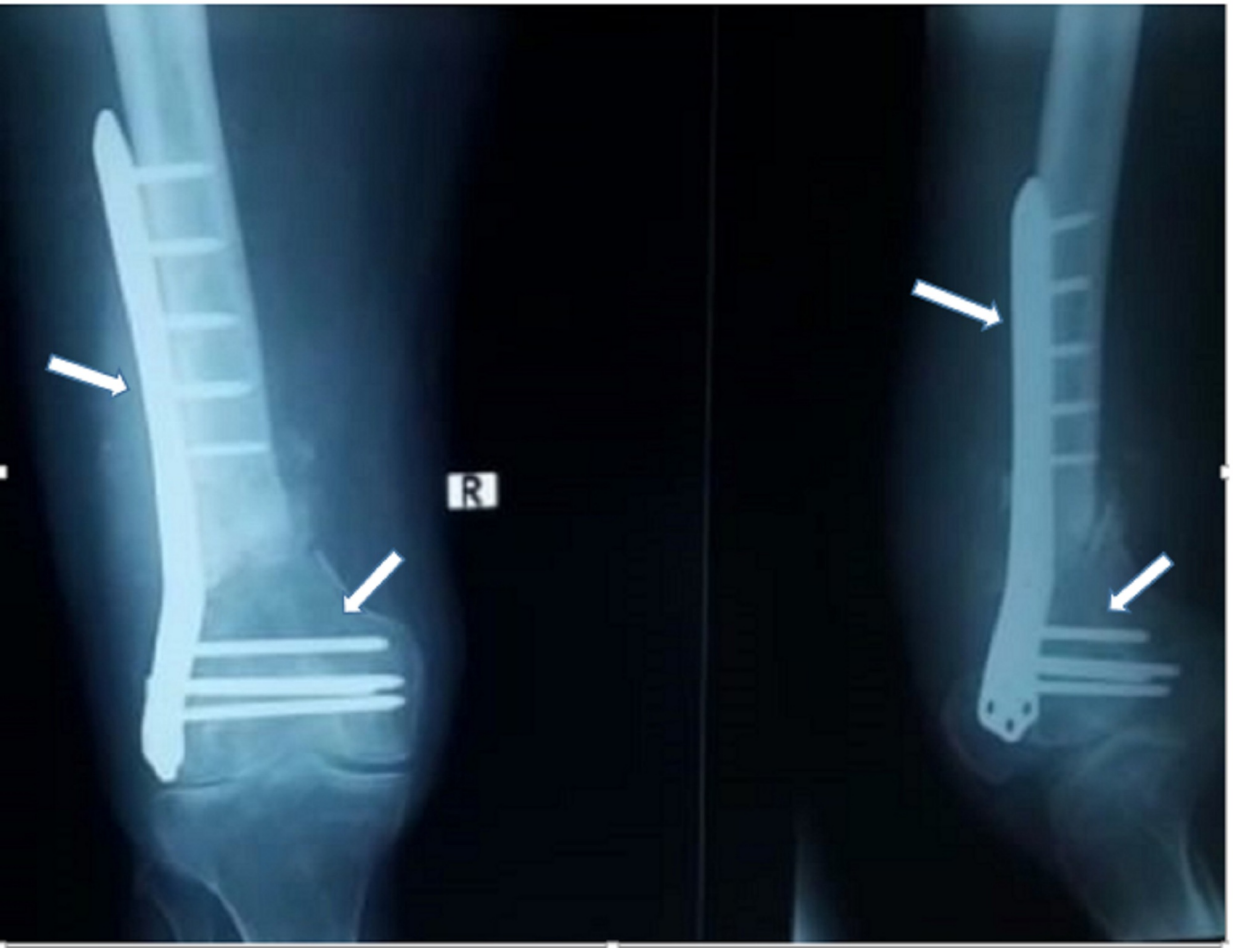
Lateral view (on right side of picture) and PA view (on left side of picture) X-rays of right distal femur showing well-reduced and aligned distal femur fracture after the surgical procedure with locking plate insertion (white arrows) and debridement of necrotic material. PA, posteroanterior
